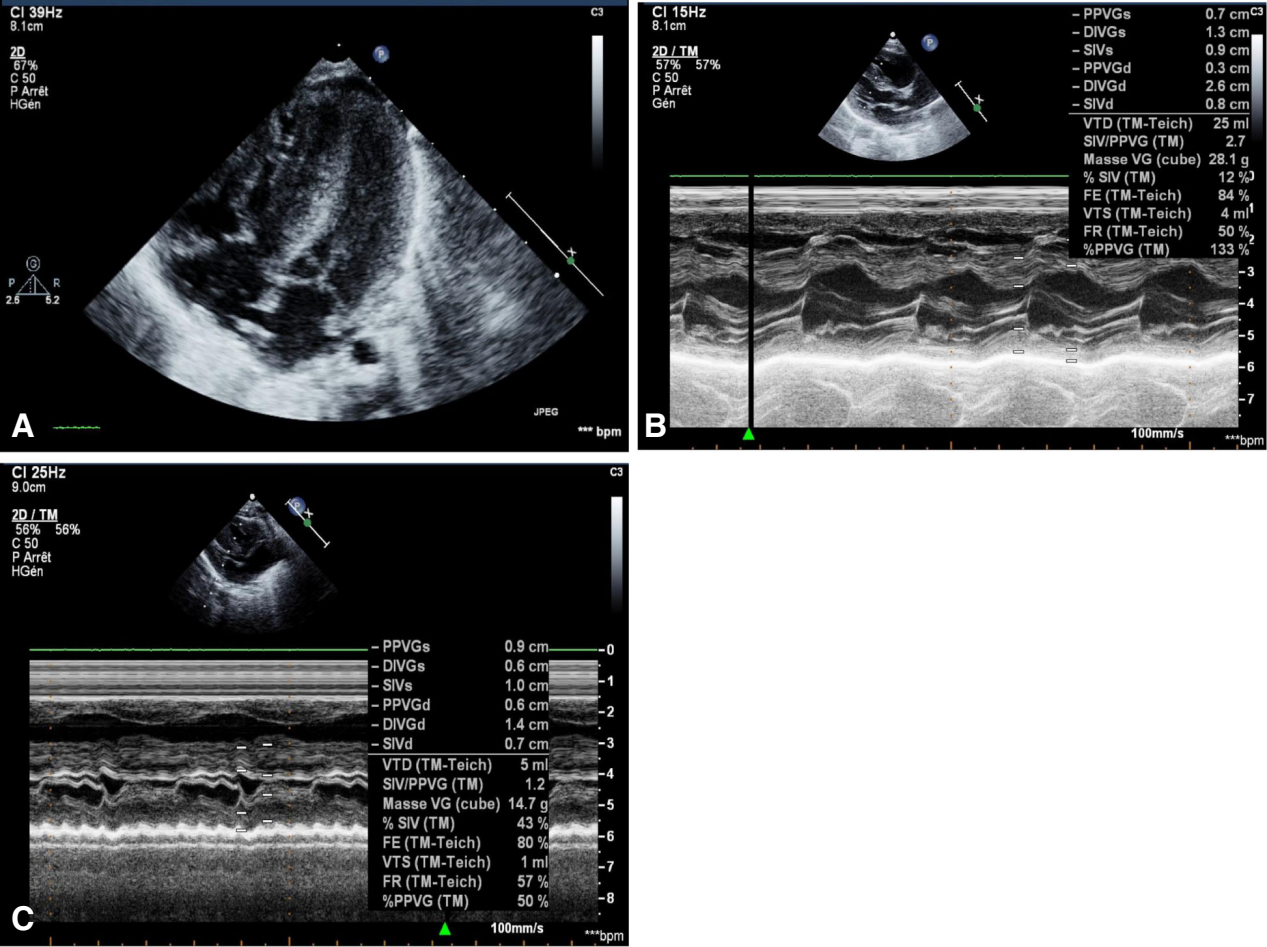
Echocardiography of the three patients. a Patient 1. Severe biventricular non-obstructive hypertrophic cardiomyopathy (interventricular septal thickness 12 mm, +5.2 Z-score) and virtual cavity in the *left* ventricle. b Patient 2. Hypertrophic cardiomyopathy predominant left (interventricular septal thickness 8 mm, +3.2 Z-score). c Patient 3. Severe hypertrophic cardiomyopathy (interventricular septal thickness 7 mm, +2.8 Z-score), with virtual cavity in the *left* ventricle and cardiac function alteration
